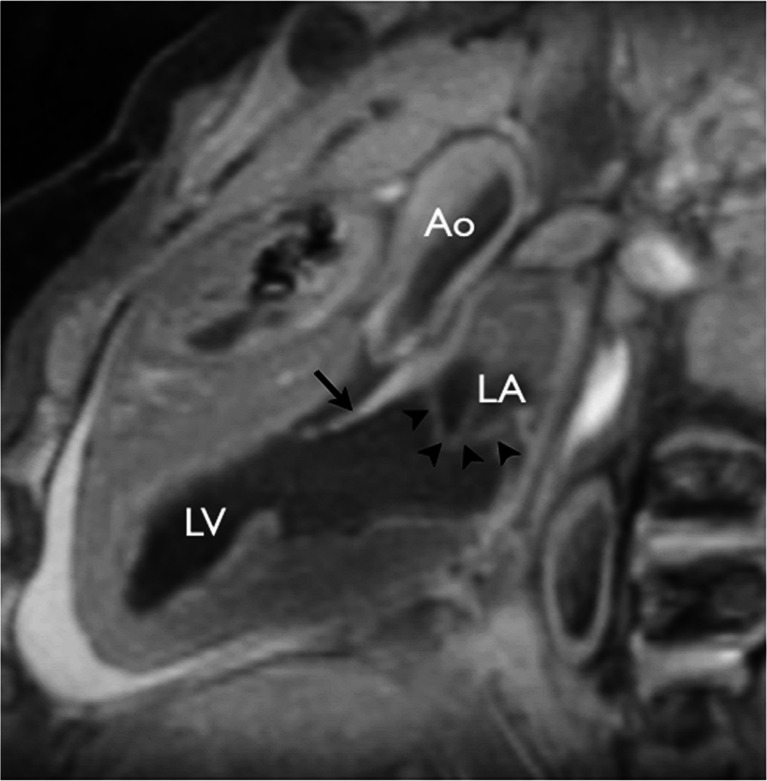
Potential overcall by cardiac postmortem MRI of cor triatriatum (*left* atrial membrane, *arrowheads*) was reported on oblique sagittal T2-weighted imaging. Long-axis view shows the *left* ventricle (*LV*), left atrium (*LA*) and aorta (*Ao*). *Arrow* indicates the open anterior leaflet of the mitral valve. Conventional autopsy of the heart was reported as normal. Reproduced with permission [14]
